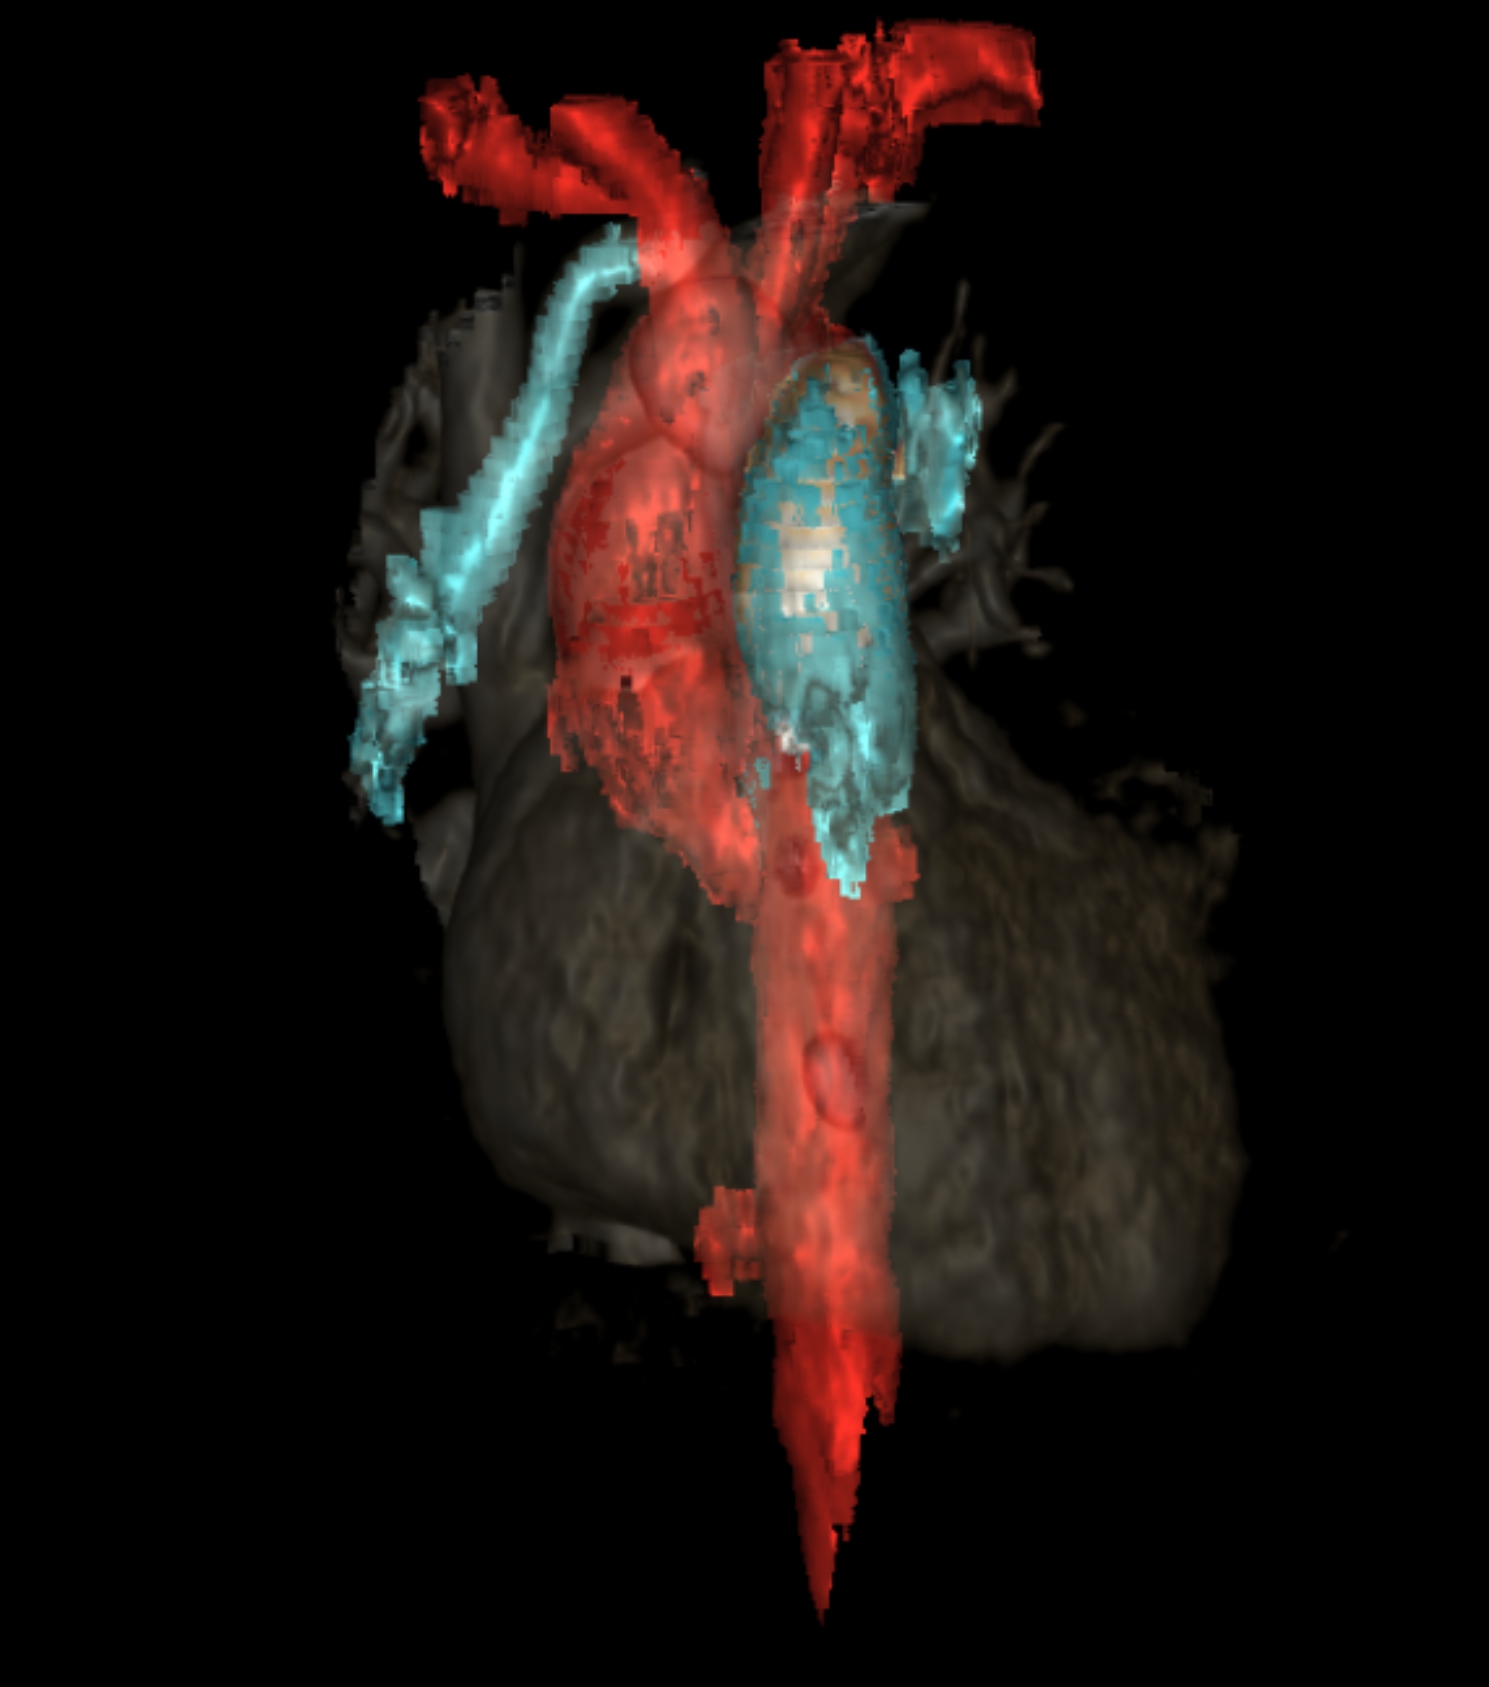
3D reconstruction of computed tomography angiography. Reconstruction showing the anomalous origin of the right pulmonary artery from the right brachiocephalic artery (indicated by the white arrow)
